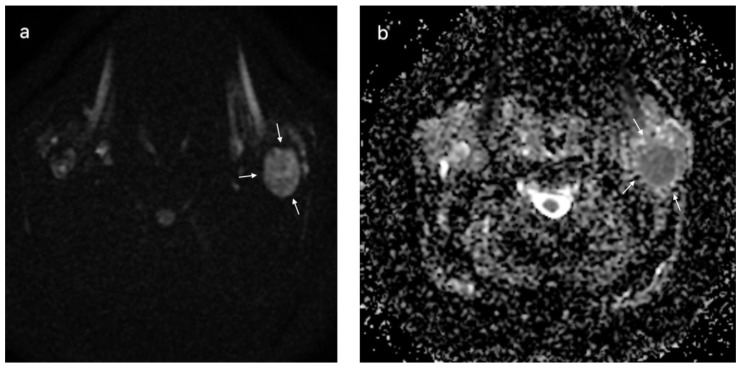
Warthin tumor of the left parotid gland (white arrows); DWI sequences (a) with ADC map (b) showing a value of 0.72 × 10^−^^3^ mm^2^/s.
